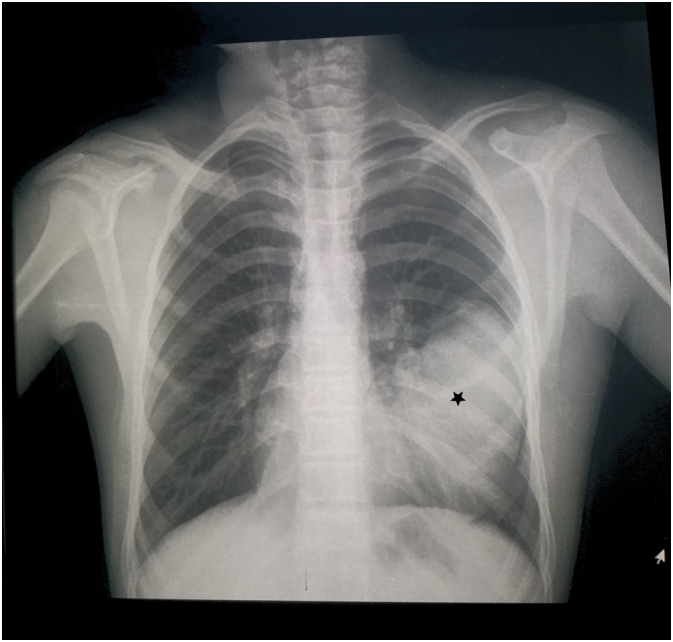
Chest X-ray on admission showing a large well-defined homogeneous radio-opacity (Black star).
